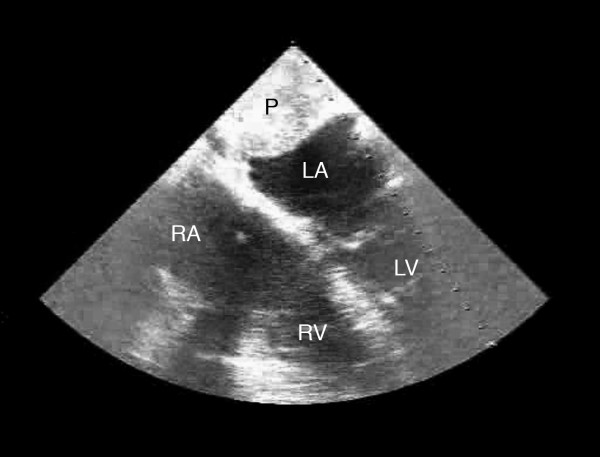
Trans-esophageal echocardiogram: TEE shows a large mass, likely extrinsic to the heart, compressing the left atrium (LA). RA denotes Right Atrium; RV denotes Right Ventricle; LV denotes Left Ventricle; P denotes the Cardiac Paraganglioma.
